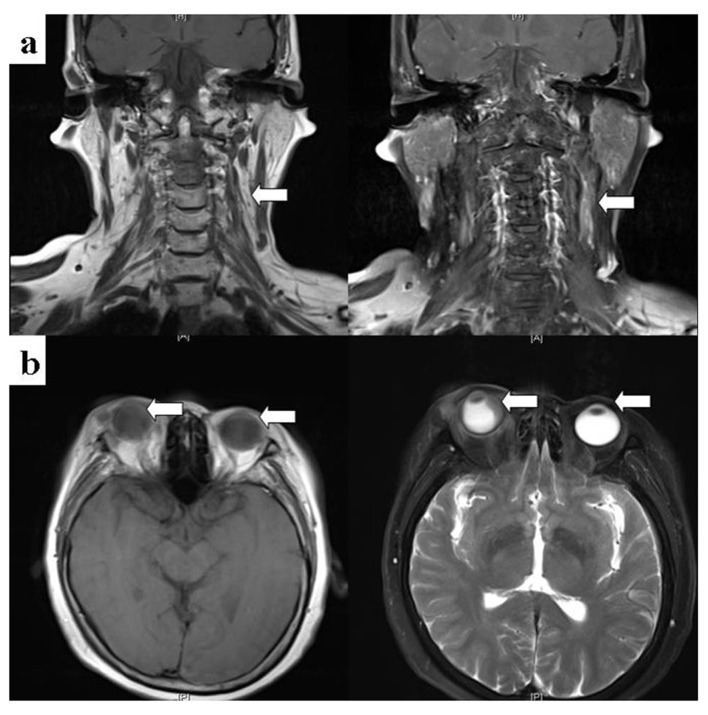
Magnetic resonance imaging (MRI) of the neck and eyeballs following two cycles of therapy. (a) Flake shadow, located in the left of the neck, was markedly enhanced following the infusion of a contrast-enhancing agent. The mass was reduced in size following treatment. (b) Both eye sockets thickened and were enhanced homogeneously. The range that was invaded by multiple myeloma reduced by approximately 60%.
